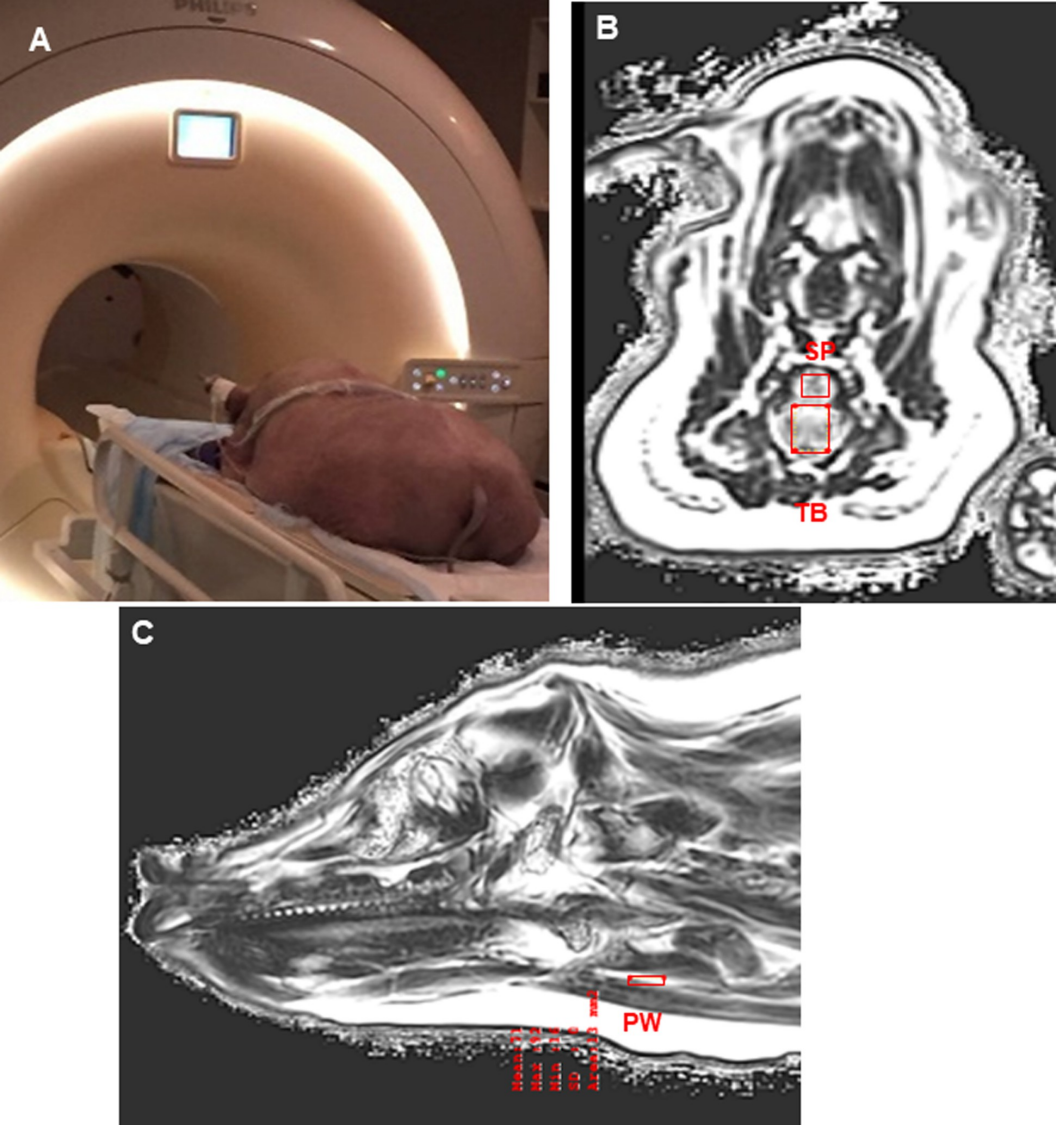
MRI imaging and fat composition measurements. A: Live MRI scanning. B: Coronal view of MRI Dixon fat water separated sequences. The two red boxes are the regions for the fat composition measurement of the tongue base (TB) and soft palate (SP). The measurements were taken in the serial 8 coronal slices from the most rostral to the most caudal with thickness of 5mm in the tongue base and soft palate. C: Sagittal view of MRI Dixon fat water separated sequences. The red box shows the region for the fat composition measurement of the pharyngeal wall (PW). The measurements were taken in the serial 3 sagittal slices from the mid-sagittal to the lateral and internal with thickness of 5mm.
